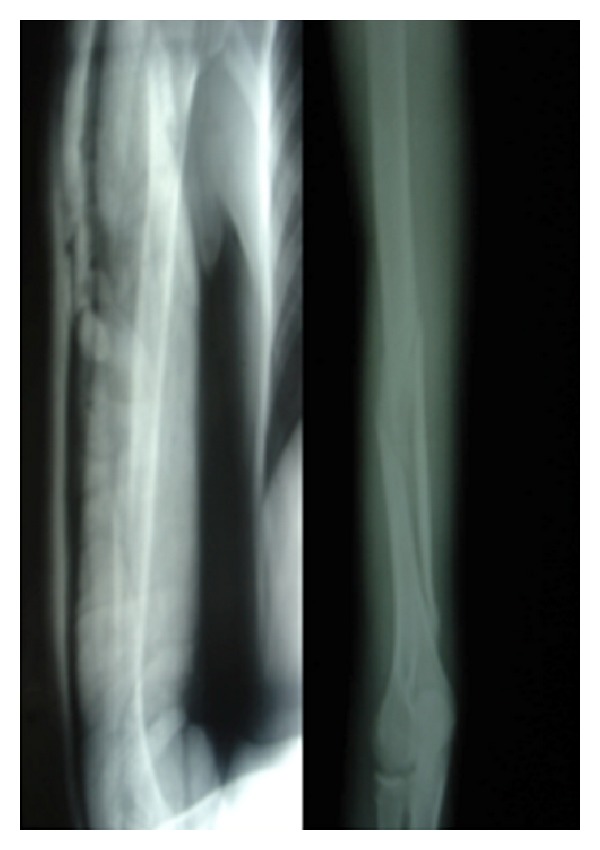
X-rays with U-splint and just after removal.
